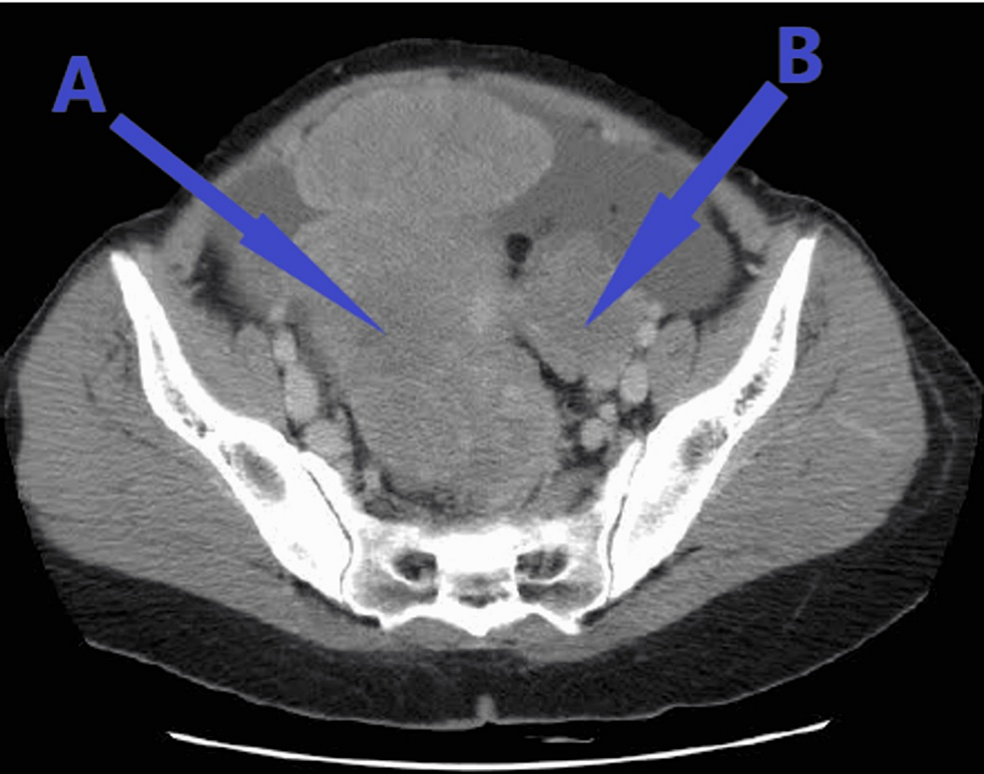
CECT of the abdomen showing extensive ascites with peritoneal carcinomatosis and bilateral heterogeneous enhancing ovarian mass. Arrows marked A and B show bilateral ovarian mass with gross ascites. CECT: contrast-enhanced computed tomography
